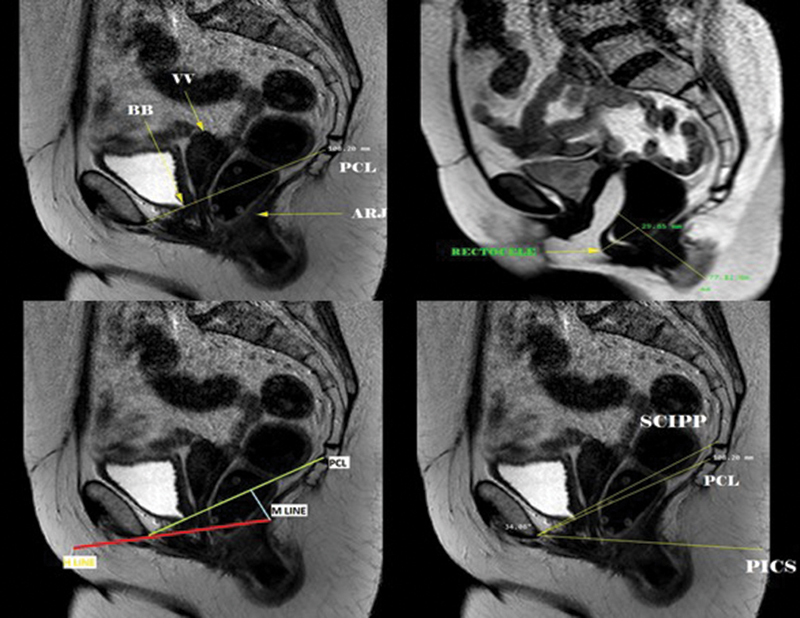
MRI in sagittal view depicting anatomical points and lines of reference (static and dynamic). Upper left (static); abbreviations: ARJ, anorectal junction; BB, bladder base; PCL, pubococcygeal line; VV, vaginal vault. Upper right (dynamic): rectocele pouch measure. Bottom left (static); red: H-line; light blue: M-line; yellow: pubococcygeal line. Bottom right (static); abbreviations: PCL, pubococcygeal line; PICS, pelvic inclination correction system line; SCIPP, sacrococcygeal inferior pubic point line.
